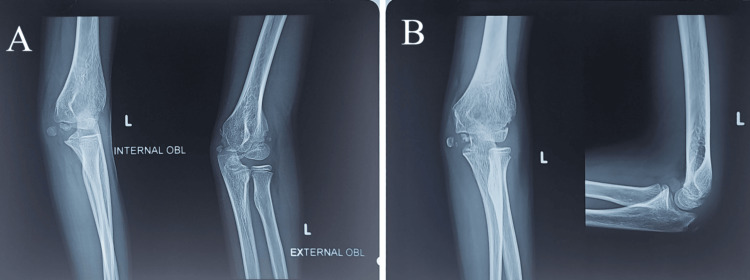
(A) Ninth-month follow-up X-ray showing internal and external oblique views. (B) AP and lateral views AP, anteroposterior
